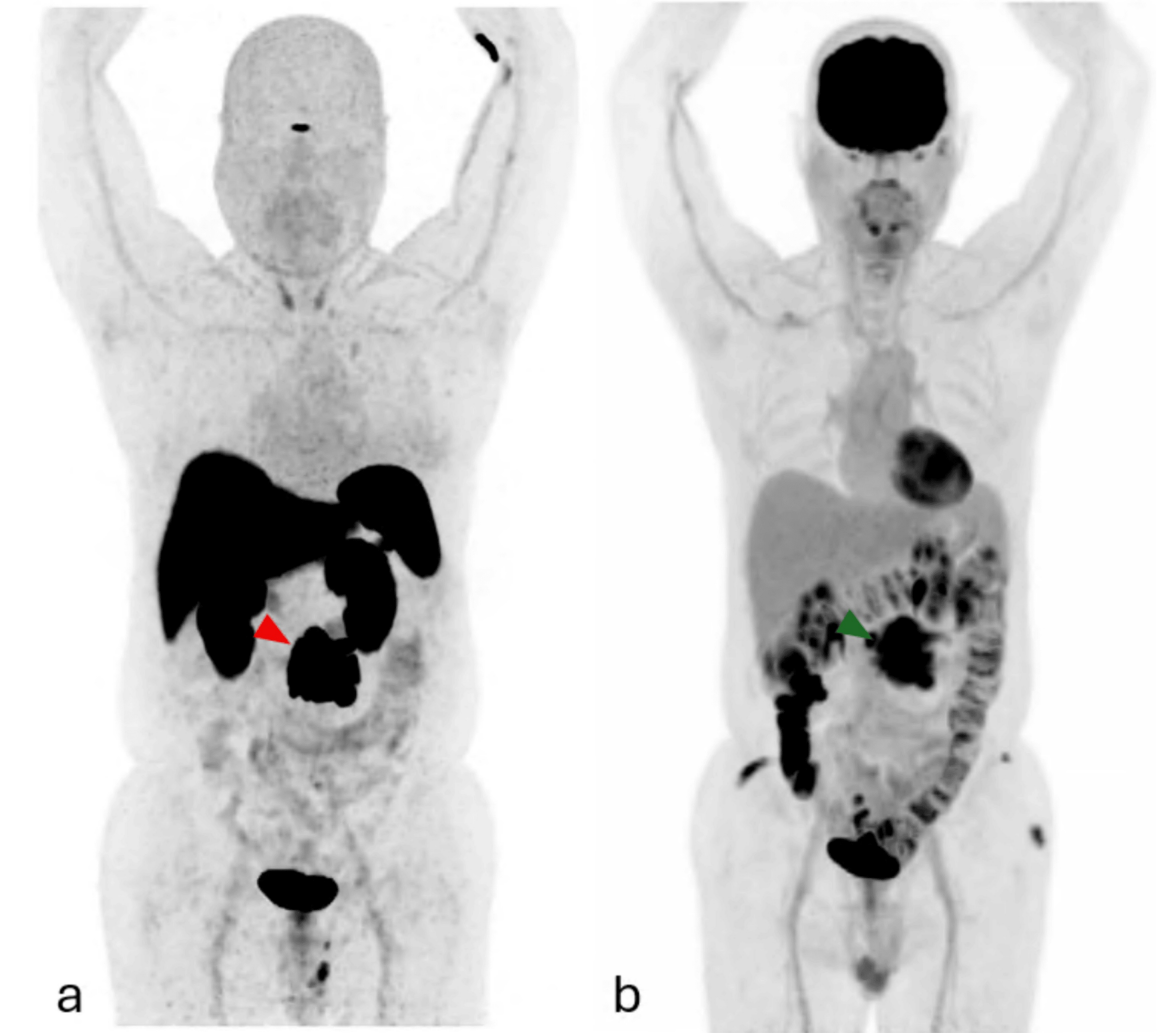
(a) MIP image from SSR PET/CT (December 2024) demonstrating intense radiotracer uptake in a nodal conglomerate in the mesenteric root (red arrowhead). (b) MIP image from ¹⁸F-FDG-PET/CT (May 2025) showing corresponding ¹⁸F-FDG uptake within the same mass (green arrowhead), indicating heterogeneous metabolic behavior MIP: maximum intensity projection, PET/CT: positron emission tomography/computed tomography, ¹⁸F-FDG: ¹⁸F-fluorodeoxyglucose, SSR: somatostatin receptor
